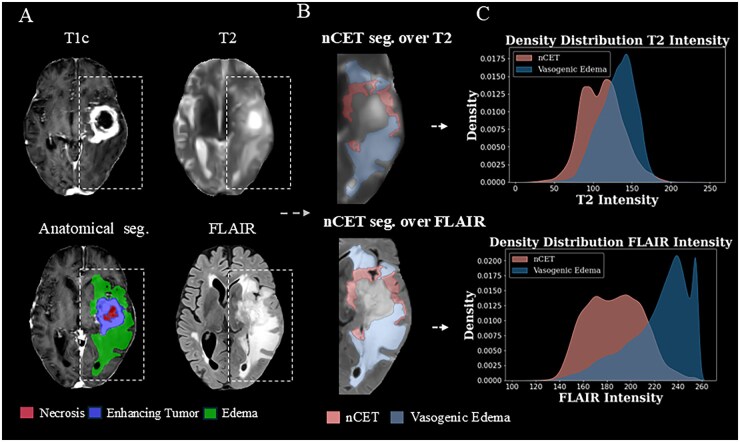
(A) Results of nCET identification obtained from T2 and FLAIR MRIs, displayed alongside the anatomical tumor segmentation. (B) Visualization of the putative nCET and vasogenic edema regions overlaid on T2 and FLAIR sequences. (C) Distribution of signal intensities in T2 and FLAIR for each region—example shown for patient OP03 from the NCT03439332 cohort.
